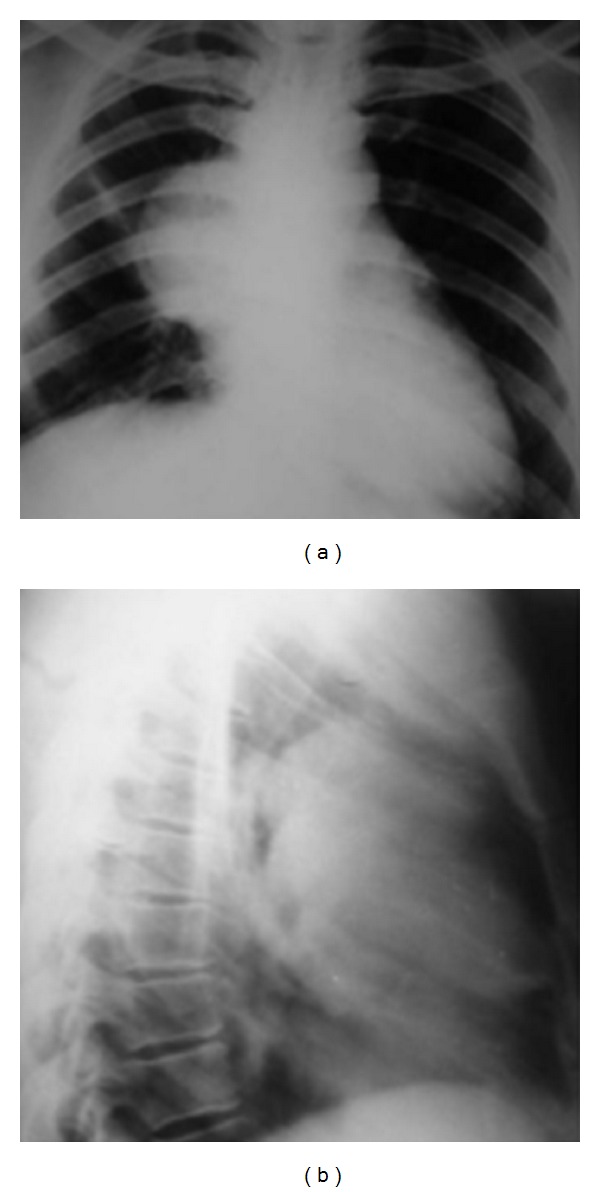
Chest radiographs, PA (a) and lateral views (b) show a well-defined mass in the anterior mediastinum.
